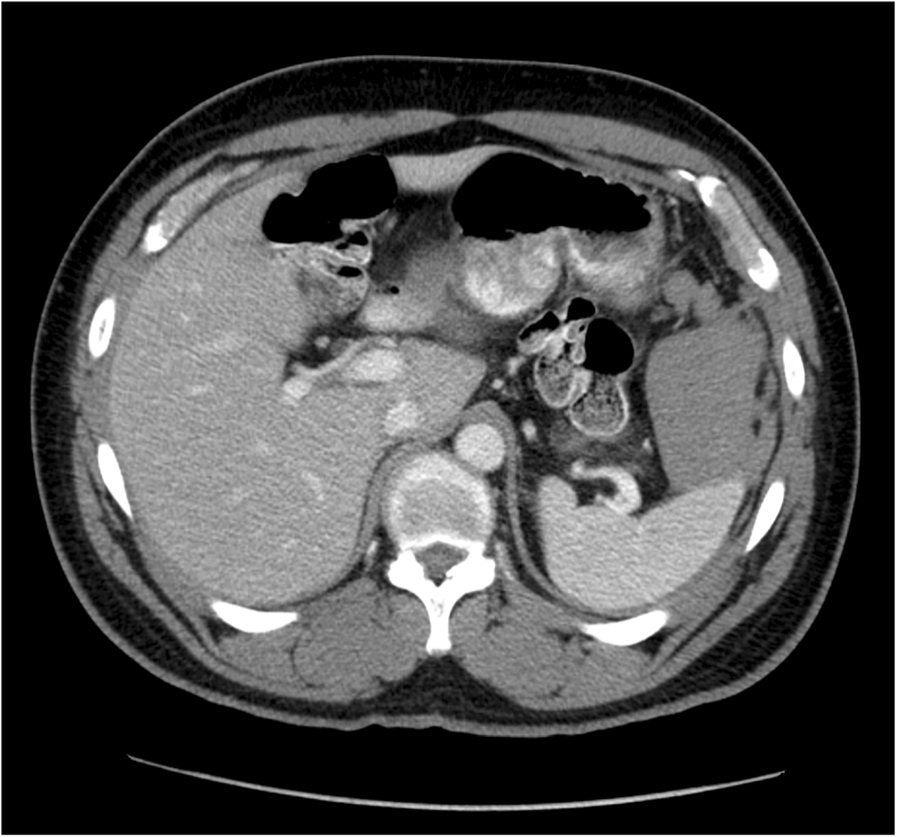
Axial, contrast-enhanced CT image demonstrates moderate hemoperitoneum in left upper quadrant centered around the spleen.
